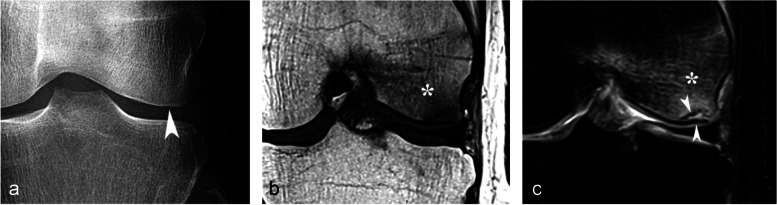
Early-stage subchondral insufficiency fracture (SIF). a Radiograph shows no significant finding apart from very subtle flattening of the condylar surface (arrowhead). b T1-weighted MR image shows moderate low signal intensity within the condyle (asterisk). c Fat-suppressed T2-weighted image shows BME-like high signal intensity more intense near the articular surface and extending to a part of the condyle without clear limits (asterisk). Note that the thin fracture line with low signal intensity is poorly visible with T1-weighting, and that with T2-weighting, high signal intensity extends within the area between the fracture and the articular surface (arrowheads). The high signal intensity in this area of the subchondral bone is an important finding to differentiate a SIF from a SIF-ON
